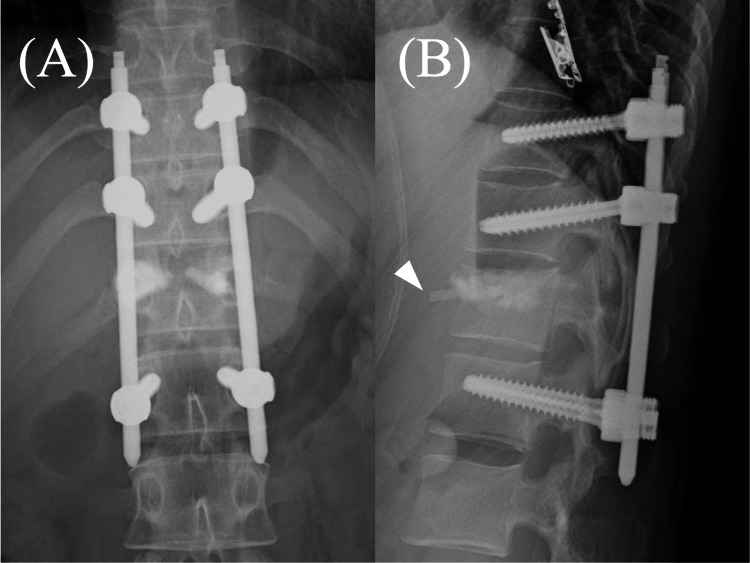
Postoperative radiographs: (A) anterior-posterior view and (B) lateral view. Anterior protrusion of hydroxyapatite blocks (arrowhead) is observed in the lateral view.
